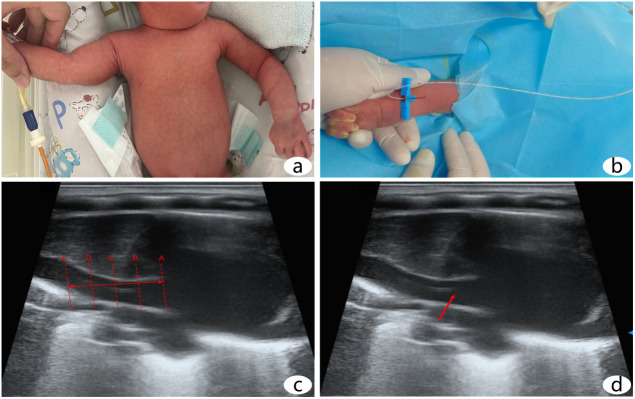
High-frequency ultrasound-guided upper limb PICC placement. a: The infant in the supine position with the right basilic vein selected; b: PICC placement guided by high-frequency ultrasound via the basilic vein (arrow indicates catheter); c: Ultrasound image of optimal catheter placement depth (A-line: superior vena cava entrance to the right atrium; B-line: 0.5 cm above the entrance; C-line: 1 cm above the entrance; E-line: 2 cm above the entrance; A-E represent the acceptable range, with B-line being ideal; < E-line is too shallow, > A-line is too deep); d: Ultrasound image of the catheter tip (arrow indicates the tip).
